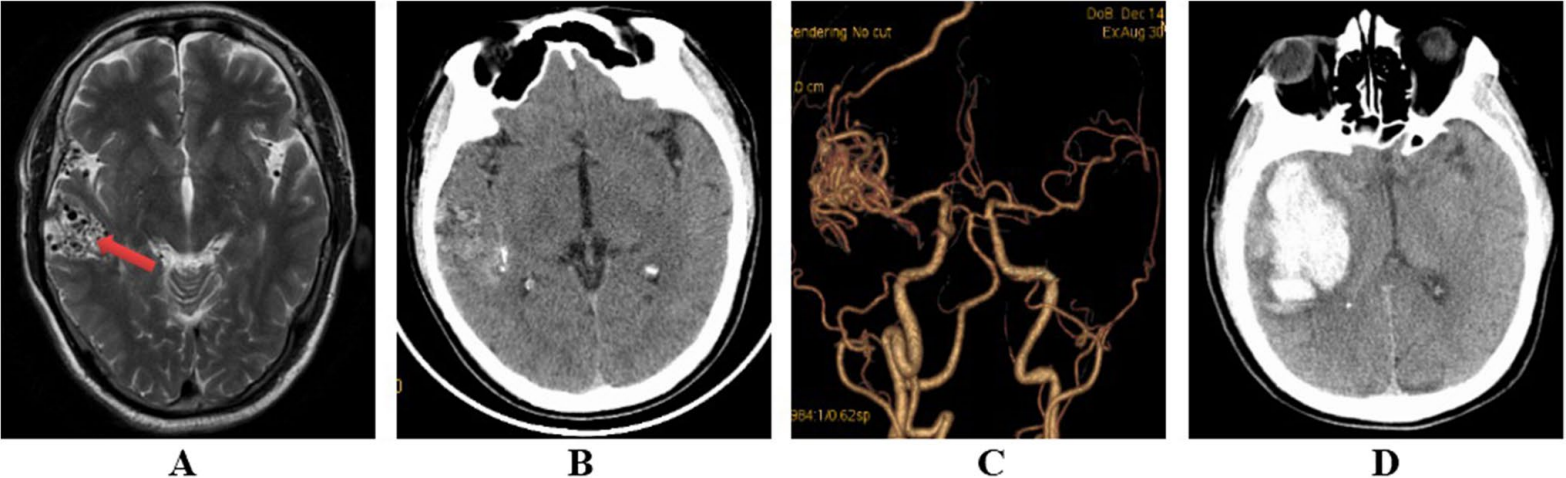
A MRI in 2018 revealed multiple tortuous and thickened blood vessels in the right temporal lobe. B, C CT angiography in 2018 showed clusters of multiple twisted and thickened vessels draining into the venous sinuses in the temporal lobe. D CT scan during the current hospital admission showed a large hematoma in the right temporal and insular lobe
